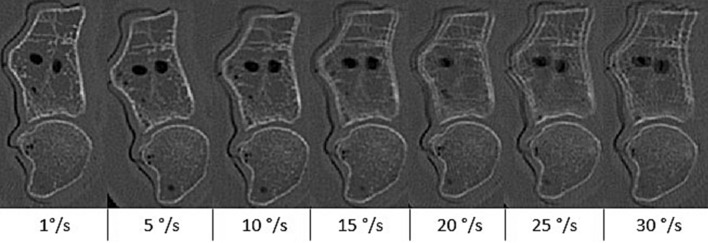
Target frame motion artefacts: CT target images at varying angular velocities. Note the shift from a blurring to a ghosting artefact at speeds greater than ten degrees per second.
